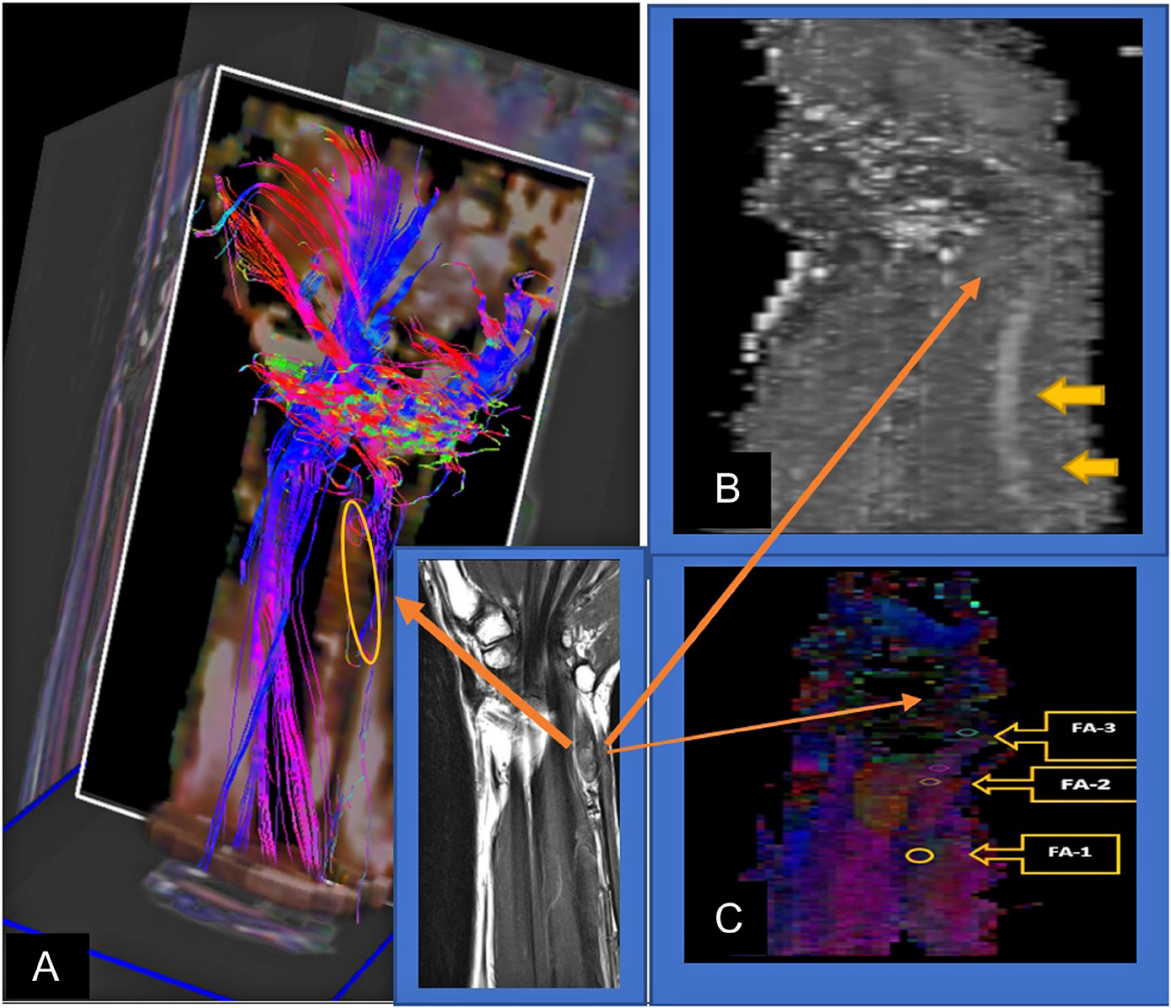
(A) (Inset) T1-weighted reference MR image of the ulnar nerve. Coronal color-coded map shows ulnar nerve (left arrow, eclipse marker). The direction of maximum diffusivity is mapped with triple colors—red, green, and blue color coding. (B) The ADC map confirms the tracked ulnar nerve fiber showing the high signal intensity of the normal caliber (right top arrow). (C) The FA map shows the tracked ulnar nerve fiber with decreasing values of the mean FA values toward the soft tissue lesion at the distal forearm—FA1 (0.96), FA1 (0.86), and FA3 (0.79).
